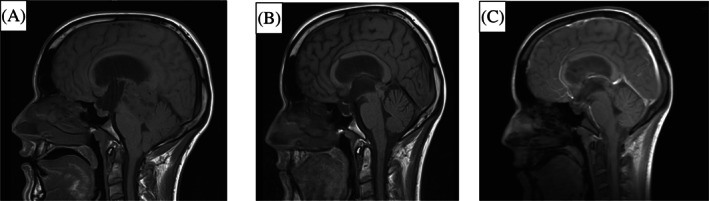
MRI T2 images of the case evolution. (A) Moment of diagnosis, (B) post NC, and (C) post WVR. NC, neoadjuvant chemotherapy; WVR, whole‐ventricular radiotherapy
